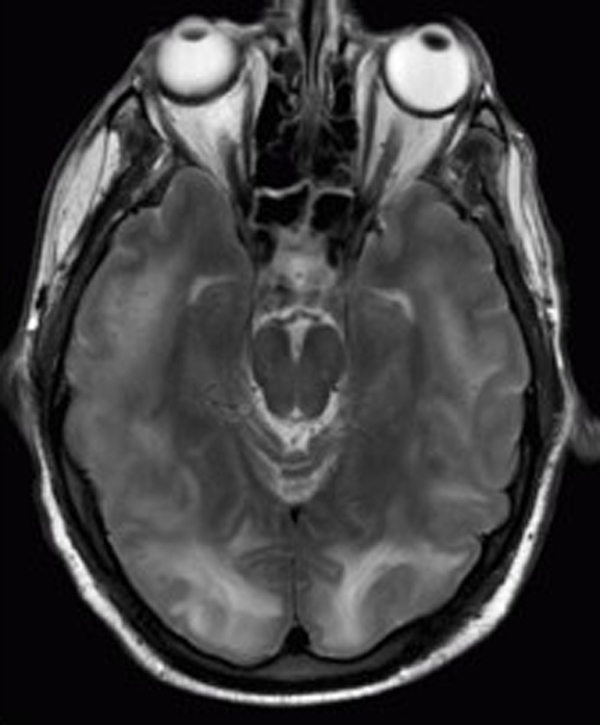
Magnetic resonance image showing multiple cortico-subcortical areas of hyperintense signal involving the occipital and parietal lobes bilaterally and pons
